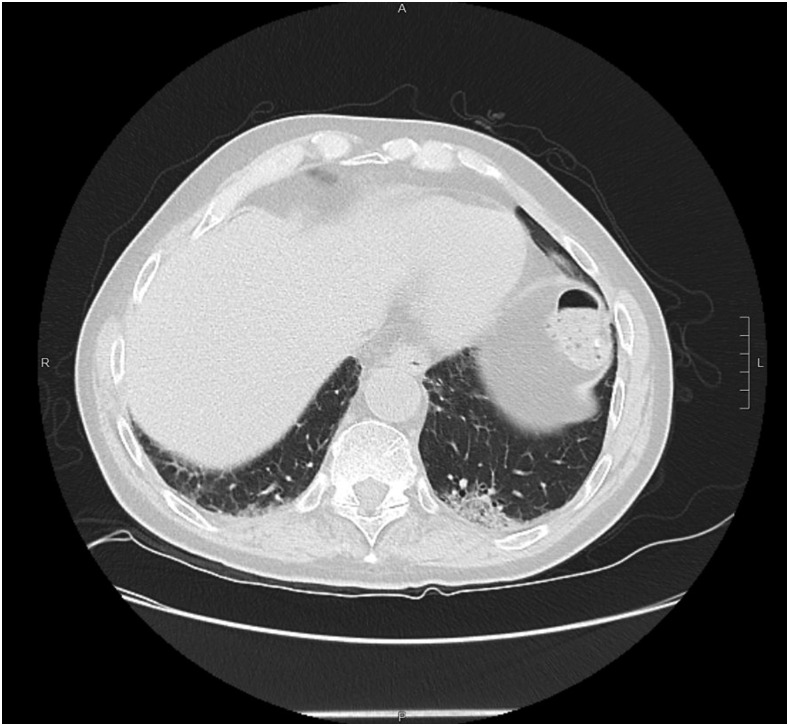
Chest CT showing a mixed density opacity in the left lower lobe.
